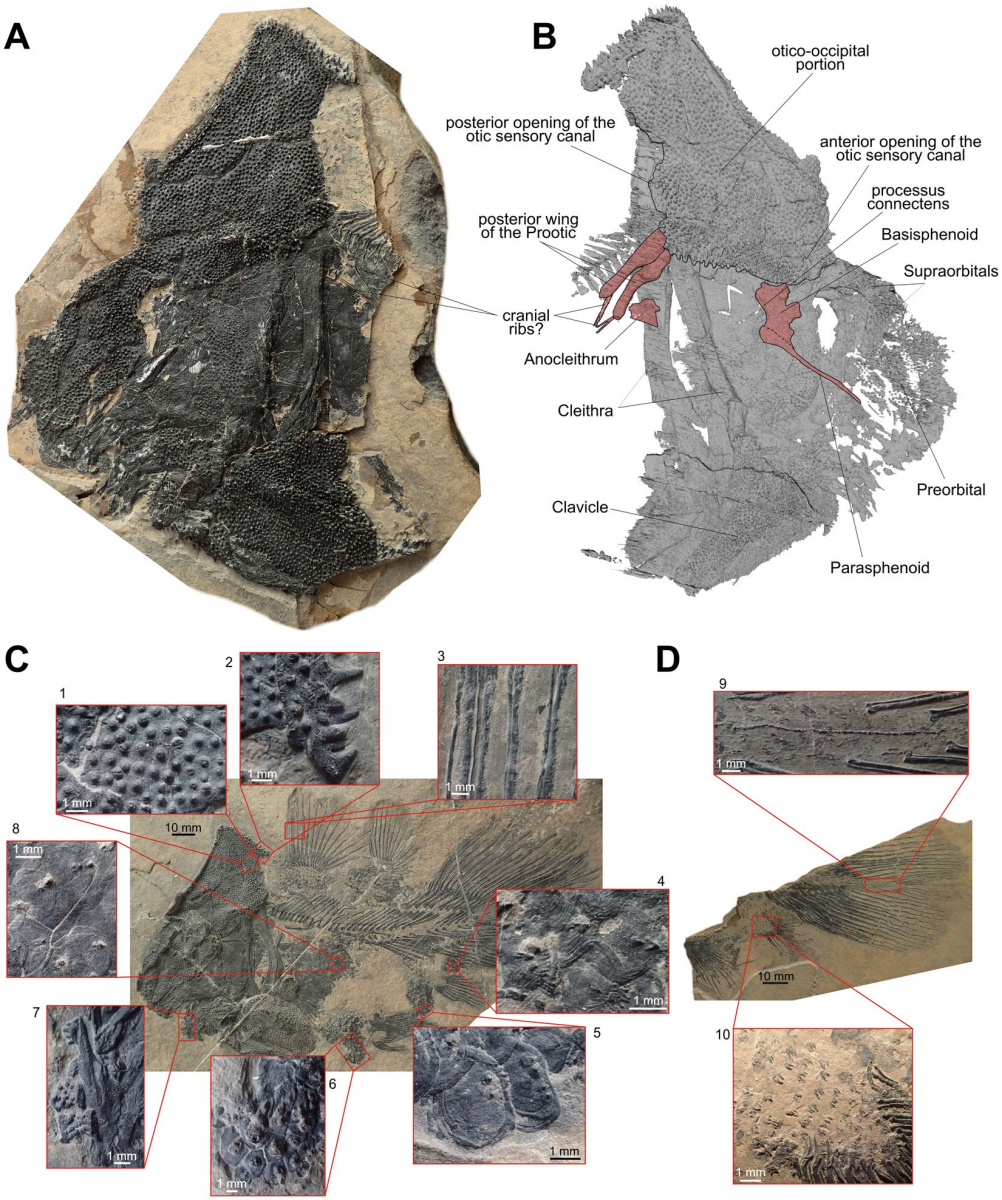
Osteological details of the new coelacanth *Foreyia maxkuhni* gen. et sp. nov. (A) Photo and (B) surface CT reconstruction of the skull of the paratype (PIMUZ A/I 4372). (C) Tubercles and denticles in the Holotype (PIMUZ A/I 4620) and (D) in the paratype (PIMUZ A/I 4372). 1, tubercles on the skull roof. 2, large spine-like tubercles on the posterior margin of the otico-occipital shield. 3, denticles on the fin rays of the first dorsal fin. 4, scales with denticles from the ventral margin of the caudal peduncle. 5, scales with denticles from the anal region. 6, scales with denticles from the belly region. 7, toothed coronoid bones. 8, scales with denticles from the flank. 9, supplementary caudal fin lobe with spiny scales. 10, Scales with denticles from the lobe of the anal fin.
